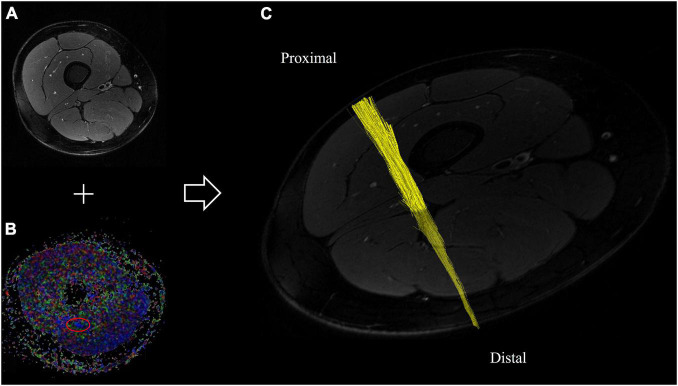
Automated segmentation of the sciatic nerve based on diffusion-weighted and T2-weighted imaging. (A) T2-weighted cross-sectional image of the right thigh, and (B) corresponding fractional anisotropy map. The sciatic nerve is delineated in red. (C) Reconstructed fiber tracts of the sciatic nerve based on diffusion-tensor imaging of the proximal thigh.
